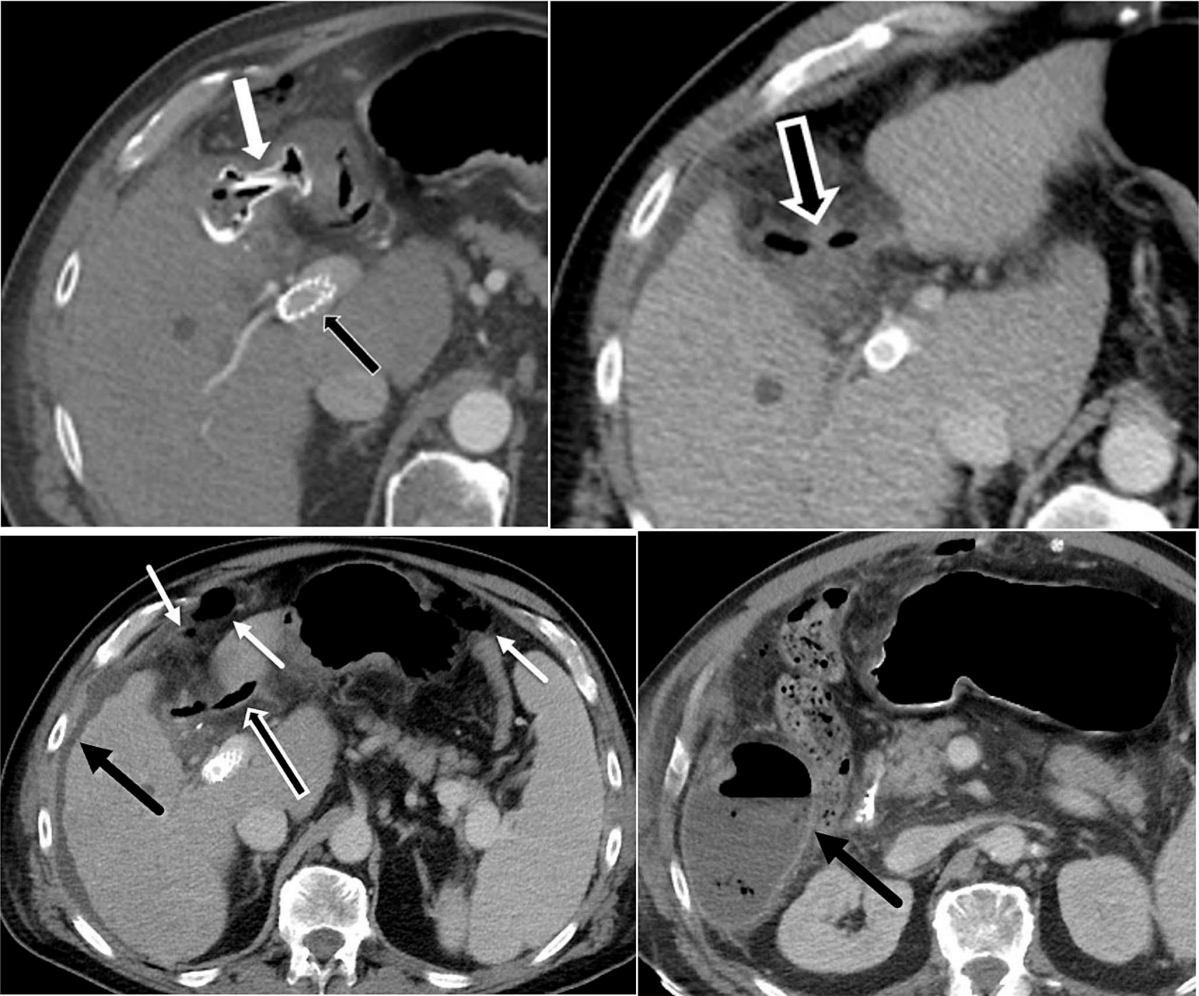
58 year-old cirrhotic male patient with acute on chronic cholecystitis complicated by portal hypertension s/p TIPS procedure. A. Contrast-enhanced axial CT image demonstrates LAMS (white arrow) placement between stomach and gallbladder with a pigtail catheter across LAMS facilitating anchorage, and TIPS (black arrow). B. One month later, interval laparoscopic cholecystectomy has taken place with removal of Axios stent. Contrast-enhanced axial CT demonstrates visualization of a residual fistulous tract (black arrow). C. A few days later, contrast-enhanced axial CT demonstrates persistent fistulous tract (outlined black arrow) leading to accumulation of free air (white arrows) and fluid in the upper and mid abdomen (black arrow). D. Three weeks later, contrast-enhanced axial CT image demonstrates interval development of right subhepatic abscess (black arrow)
